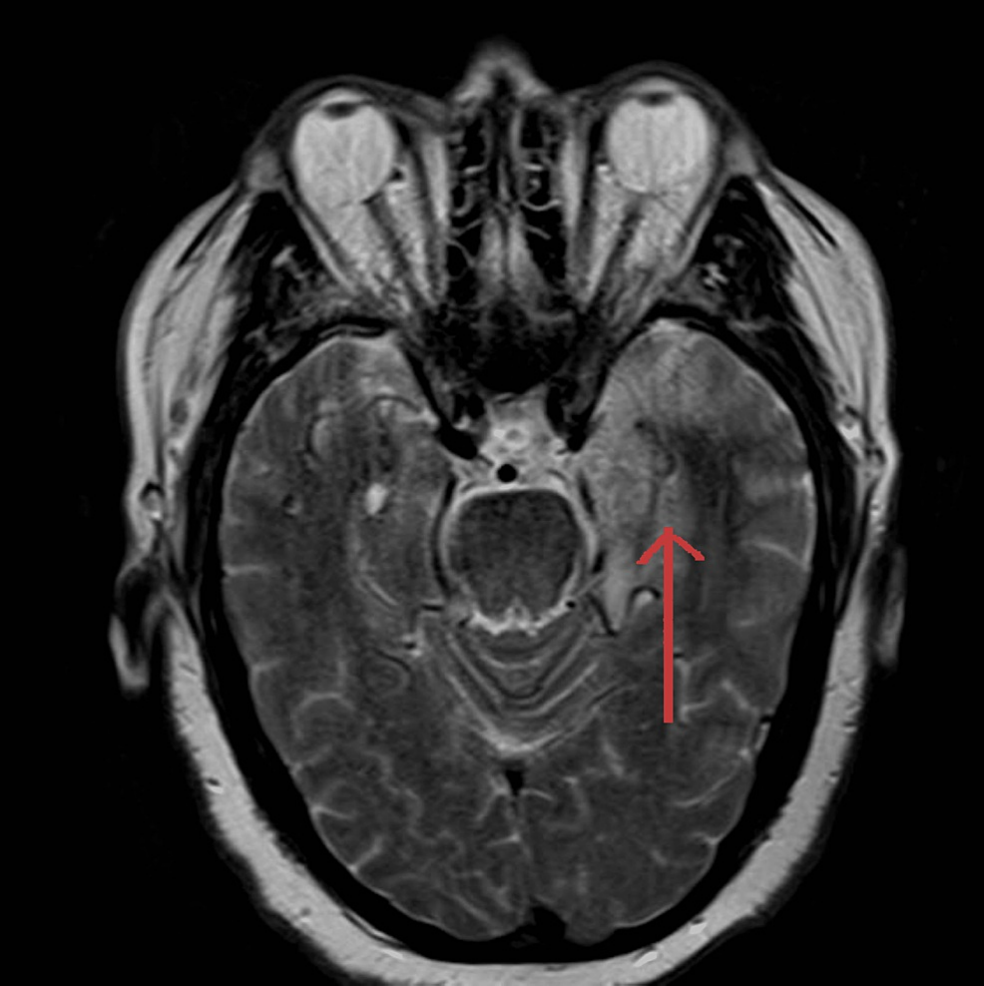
MRI scan, T2-weighted imaging phase Abnormal high signal on T2 involving the left insular cortex and left temporal lobe. No intra- or extra-axial hemorrhage was seen.
